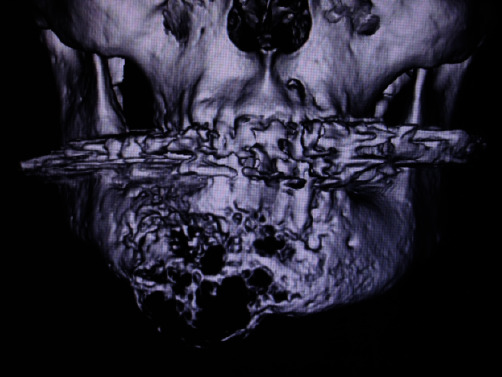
3D-CT showing wide bone destruction of the right anterior mandible, suggesting tumor extension beneath the mucosa and skin from the cortical bone. Small bone fragments have been pushed away to the outside.
